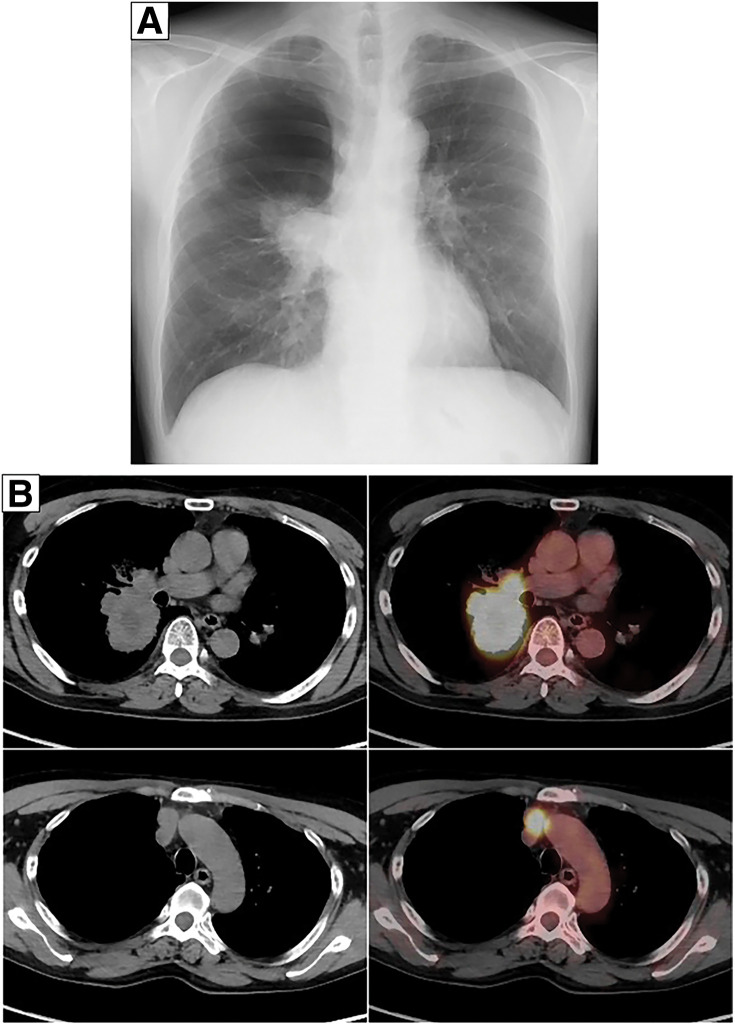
Representative radiological images. (A) Chest radiograph showing a mass shadow in the right hilar region. (B) Chest CT and fluorine-18-fluorodeoxyglucose PET showing a 55 × 42-mm mass extending from segment 2 to segment 6, with a maximum standardized uptake value of 17.20 in the upper panels. The lower panels show enlargement of the prevascular (#3a) lymph node with maximum standardized uptake values of 14.55.
